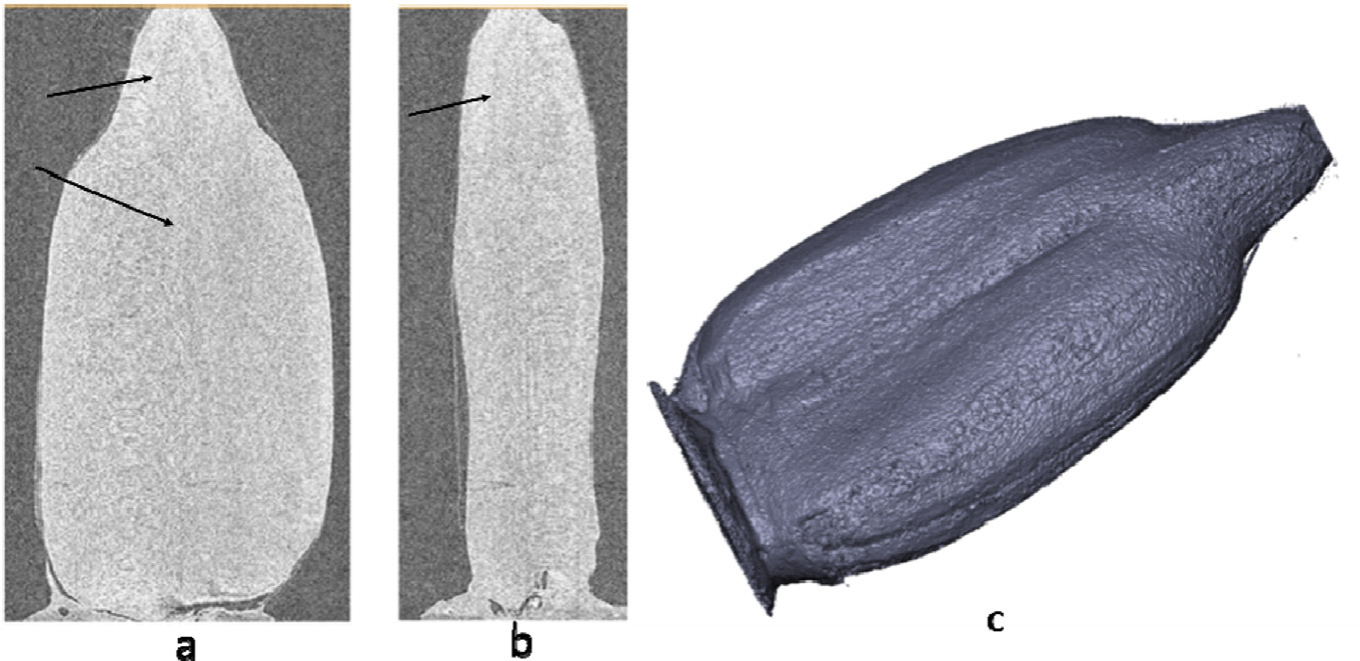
Transverse section (a) and longitudinal section (b) of sunflower kernel and main view of the sunflower kernel obtained from x-ray microtomography (c).
